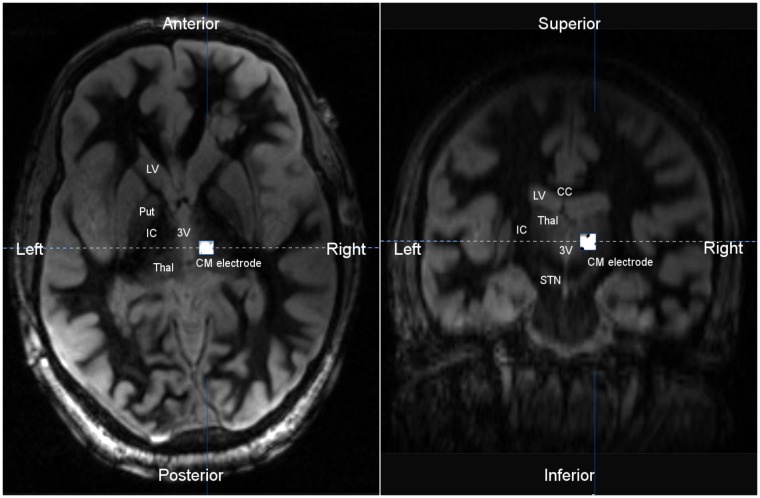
Axial (Left) and coronal (Right) T2 MR image co-registered with post-operative CT scan. The implanted centromedian (CM) depth electrode was localized to the central thalamus. The location of the depth electrode was verified by co-registration of a post-operative CT scan, with CM depth electrode artifact, and a pre-operative MRI. T2 imaging shows the thalamus bordered by the third ventricle (3V) and internal capsule (IC). LV, lateral ventricle; Put, putamen; Thal, thalamus; IC, internal capsule; CM, centromedian thalamus; STN, substantia nigra; CC, corpus callosum.
